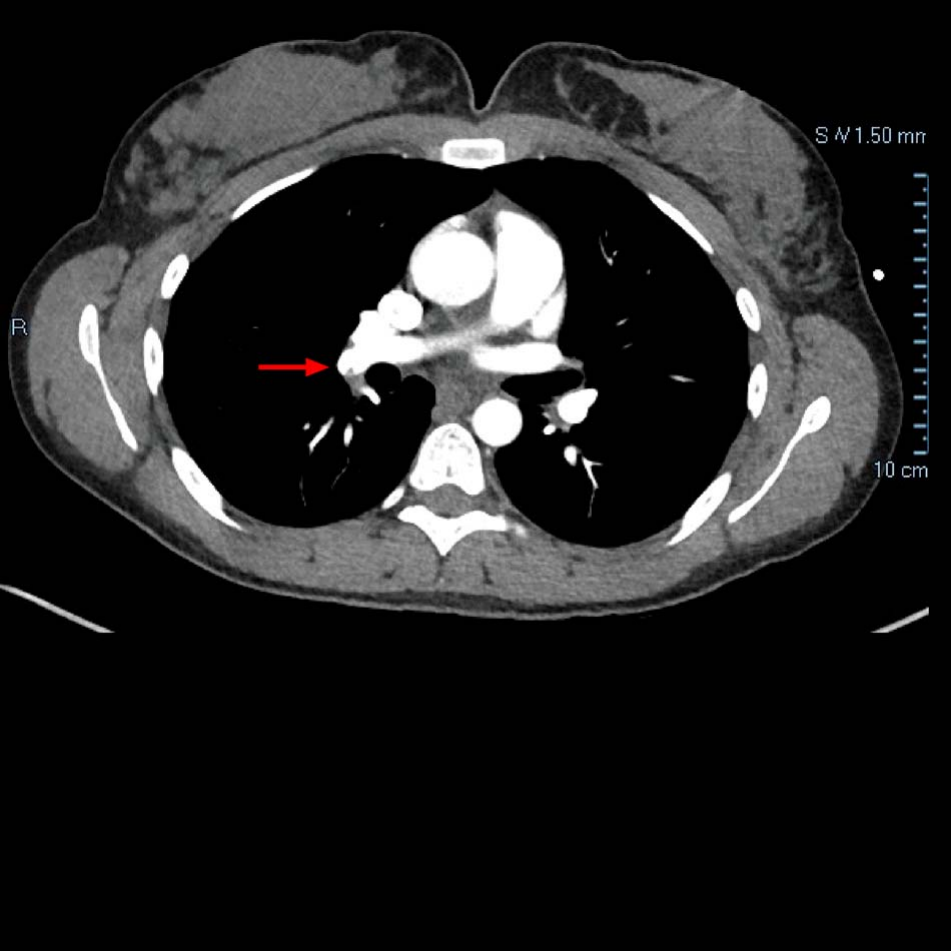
Chest CTA demonstrating filling defect within the right lower lobar pulmonary arterial vasculature consistent with pulmonary embolus as indicated by the red arrow.
